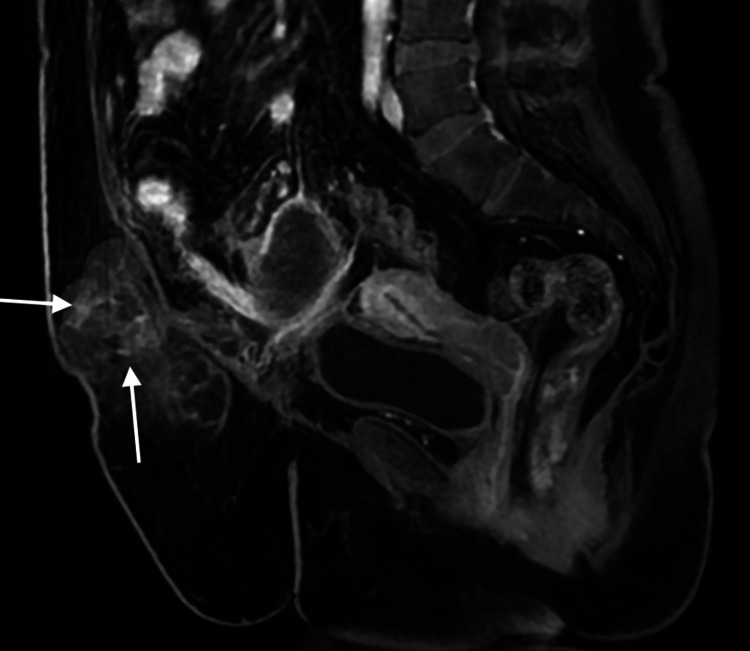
MRI of the abdomen and pelvis in a sagittal T1 fat sat sequence, post IV contrast administration. IV enhanced fat-suppressed T1WI shows enhancement of lesion, mostly at the internal septae (arrows). The craniocaudal dimensions of the hernial sac are better demonstrated in this sagittal sequence.
